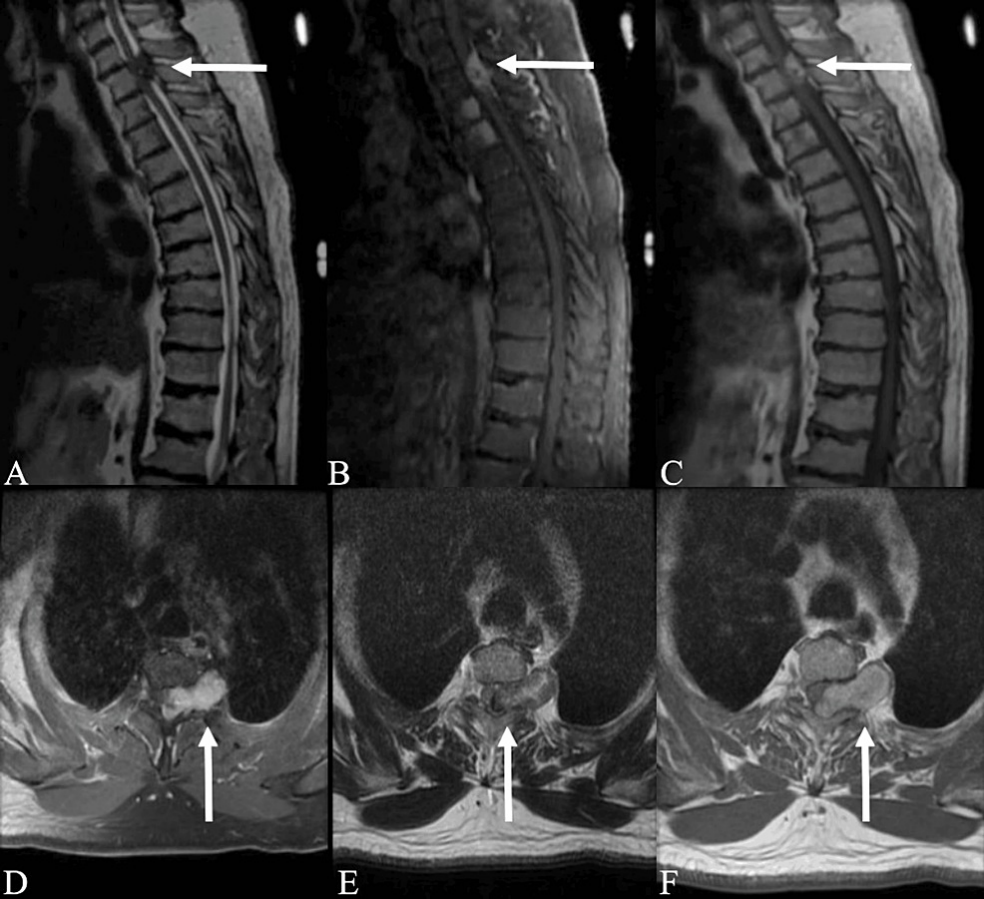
Preoperative MRI reveals ventral T2-3 left dumbbell-shaped spinal epidural melanoma involving thoracic cavity. The lesion appears hyperintense on sagittal and axial T1-weighted imaging (A: sagittal; D: axial) and hypointense on sagittal and axial T2-weighted imaging (B: sagittal; E: axial). Gadolinium-enhanced MRI reveals homogenous enhancement for the melanoma lesion (C: sagittal; F: axial).
